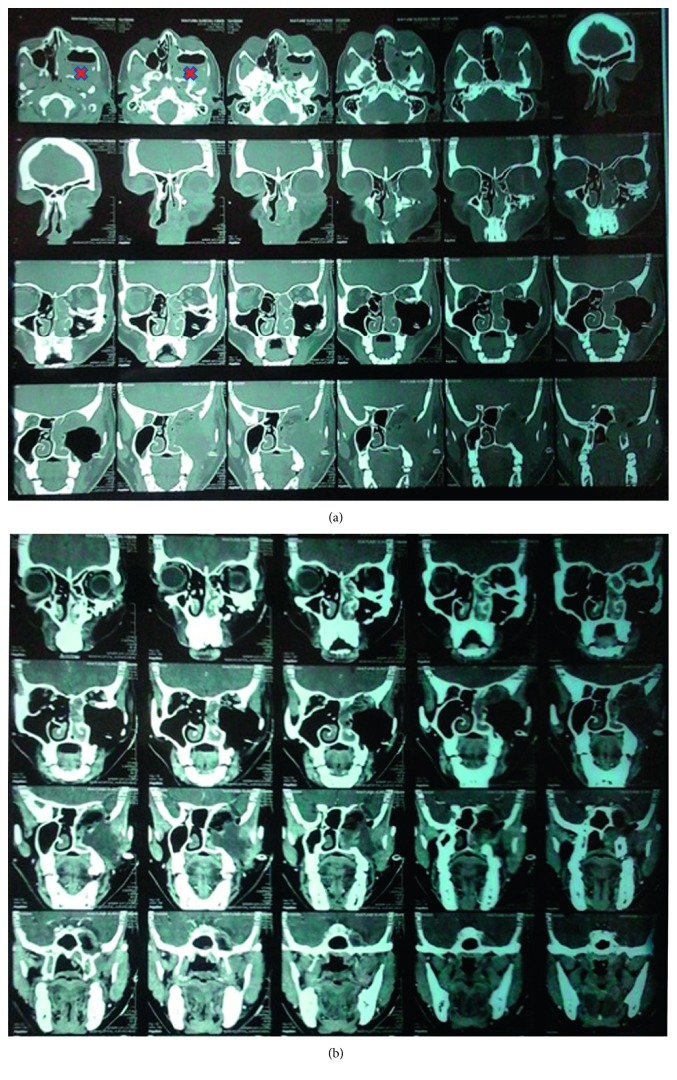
(a) Preoperative CT showing the tumor (^∗^) present in the posterior part of the left maxillary sinus and the left nostril. (b) Postoperative CT.
